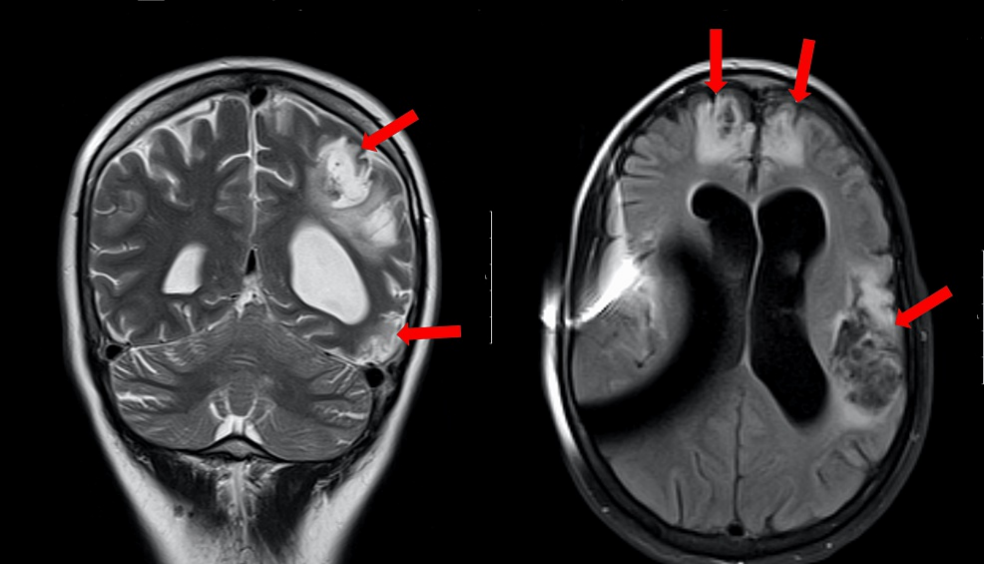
Cerebral MRI showed ischemic lesions in different evolutionary stages in both ACA and MCA territories. ACA, anterior cerebral artery; MCA, middle cerebral artery
